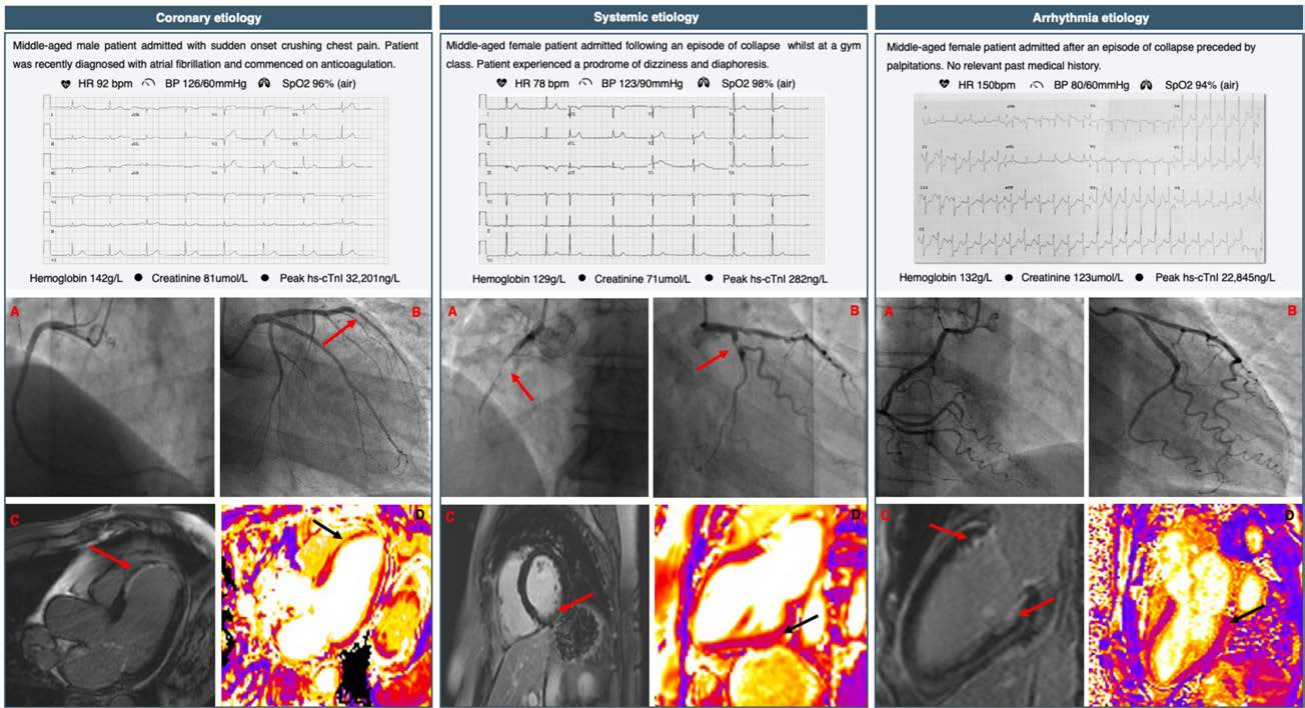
Exemplar cases and imaging studies according to cause of type 2 myocardial infarction. Patients with 3 differing causes of type 2 myocardial infarction showing clinical presentation data; study imaging, including invasive coronary angiogram; and cardiac magnetic resonance images. Coronary cause case: Invasive coronary angiogram (A) with evidence of coronary embolus with occlusion of the mid left anterior descending coronary artery (B). Cardiac magnetic resonance showed normal ventricular size and moderate impairment in left ventricular function (ejection fraction, 48%) with evidence of near-transmural late gadolinium enhancement in the anteroseptum (C). T2 value at the site of late gadolinium enhancement was elevated at 75.6 milliseconds, indicating an acute infarct (D). Systemic cause case: Invasive coronary angiogram showed evidence of 3-vessel obstructive coronary artery disease (A and B). Cardiac magnetic resonance showed normal ventricular size and function (ejection fraction, 64%) with evidence of subendocardial late gadolinium enhancement in the inferior wall (C). T2 value at the site of late gadolinium enhancement was elevated at 49.6 milliseconds, indicating an acute infarct (D). Arrhythmia cause case: Normal invasive coronary angiogram (A and B). Cardiac magnetic resonance showed normal ventricular size and function (ejection fraction, 62%) with evidence of basal subendocardial late gadolinium enhancement affecting the inferior, inferolateral, and anterior walls (C). T2 value at the site of late gadolinium enhancement was elevated at 51 milliseconds, indicating an acute infarct (D). BP indicates blood pressure; and HR, heart rate.
